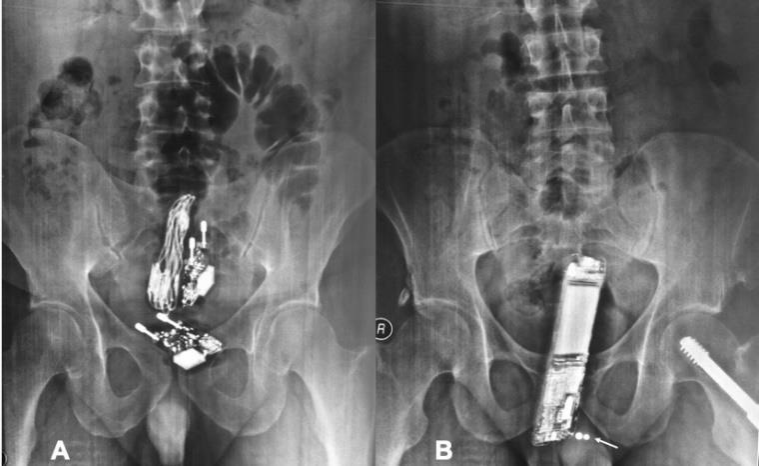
Abdominal x-rays of(A) first prisoner bearing two smartphone chargers rectally; (B) second prisoner bearing a smartphone rectally; incidental penis pearling (arrow)
